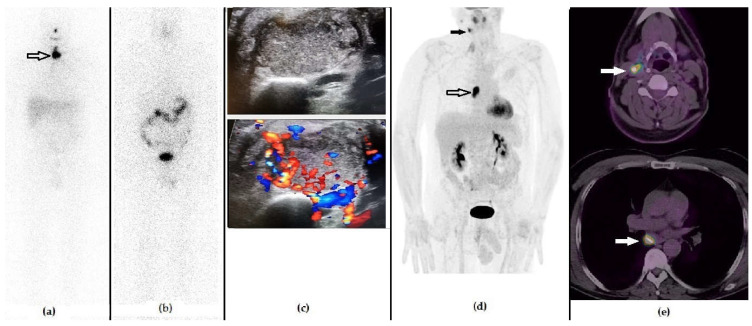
A 56-year-old male that previously underwent a thyroidectomy plus CND and 1 cycle of RAI due to Hürthle cell carcinoma (pT1b N1b). The first panel represents the post-RAI ^131^I-WBS showing the residual iodine-avid tissue in the anterior cervical region ((a), black arrow). Fifteen months after RAI, he presented with a slightly increased value of stimulated Tg serum level (i.e., 3.5 ng/mL), negative ^131^I-WBS (b) and neck US showing hypervascularized laterocervical node suspected of metastasis. (c) ^18^F-FDG PET/CT demonstrated a highly increased tracer incorporation in the suspected right laterocervical node, ((d) black arrow) also revealing metastases to the mediastinal nodes ((d), black bordered arrow), as evident in the whole body (d) and in the fused PET/CT axial images (e). The patient underwent surgery, followed by a further cycle of RAI.
